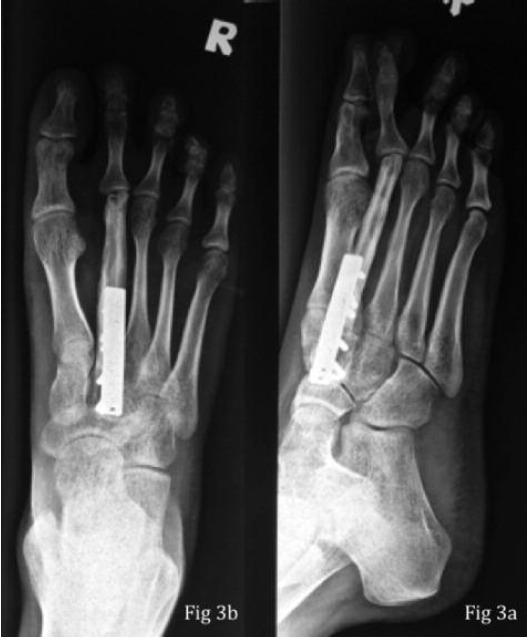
Radiograph taken at 24 months post surgery showing
